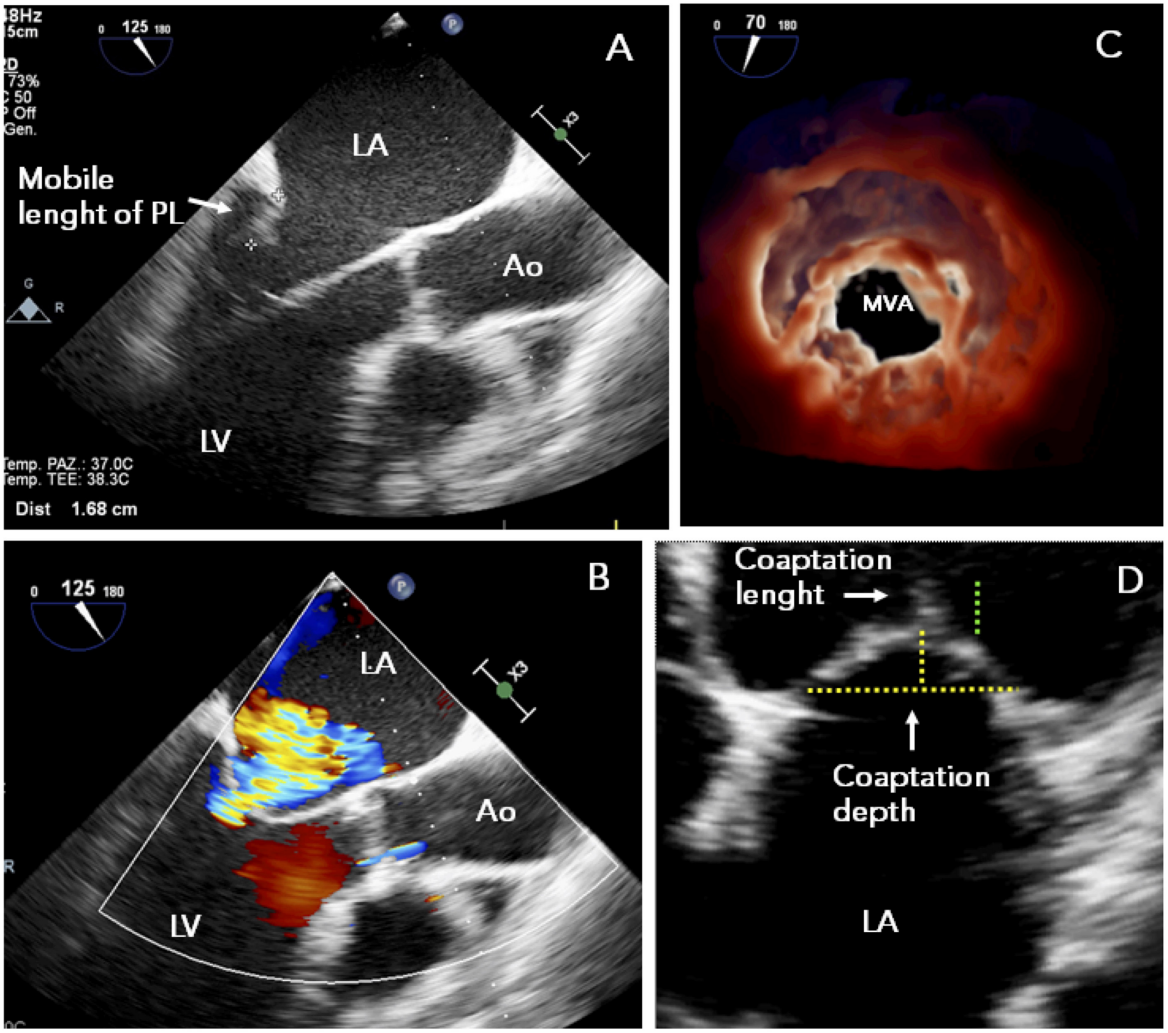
TTE study in a patient presenting with functional MR and mitral valve morphology “suitable” to MitraClip: (A) posterior leaflet mobile length ≥10 mm; (B) symmetrical tethering of non-calcified leaflets with central regurgitant jet; (C) adequate MVA (>4 cm^2^); (D) preserved leaflet coaptation. Ao, ascending aorta; LA, left atrium; LV, left ventricle; MR, mitral regurgitation; MVA, mitral valve area; PL, posterior leaflet; TEE, transesophageal echocardiography.
